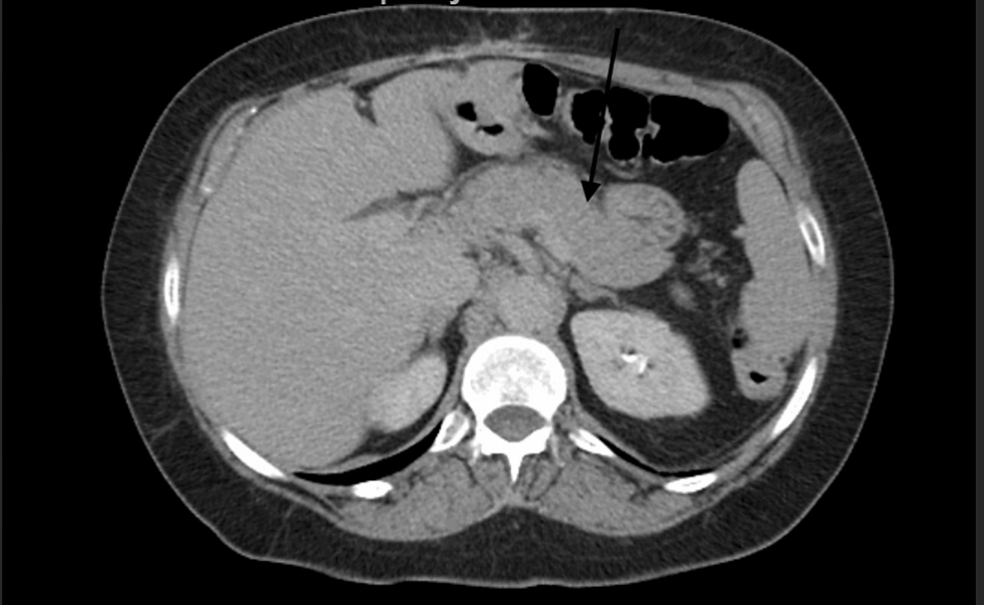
CT abdomen The black arrow demonstrates a normal pancreas.
